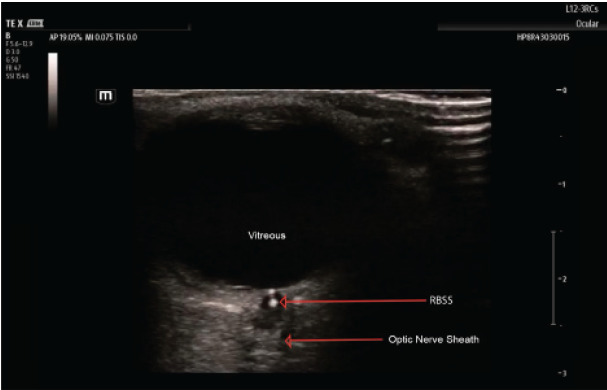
Findings on ocular point-of-care ultrasound using a linear probe demonstrate a retrobulbar spot sign (RBSS) in the transverse view, consistent with central retinal arterial occlusion.
